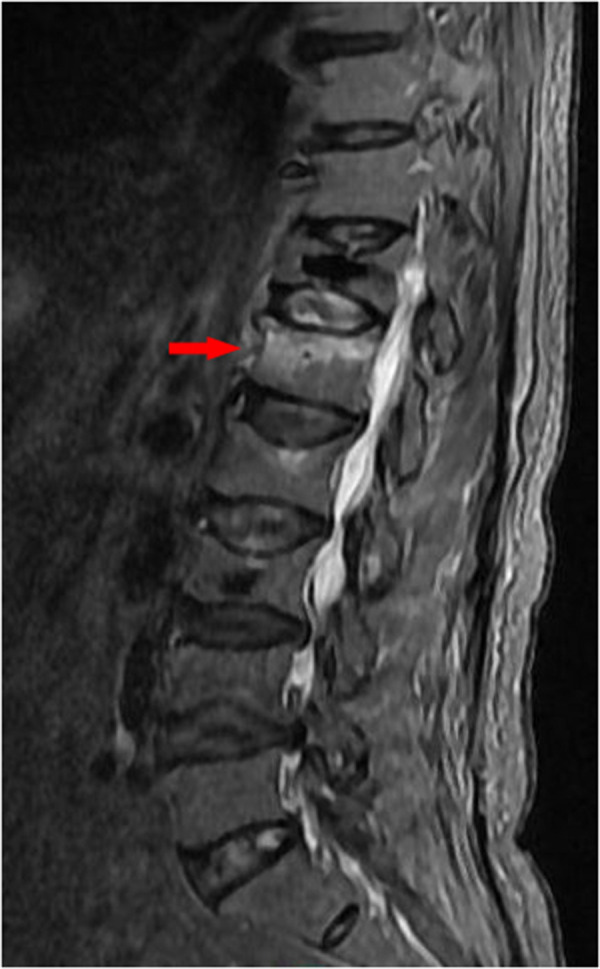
Preoperative MRI of lumbar spine suggested compression fracture of L1 vertebral body.
